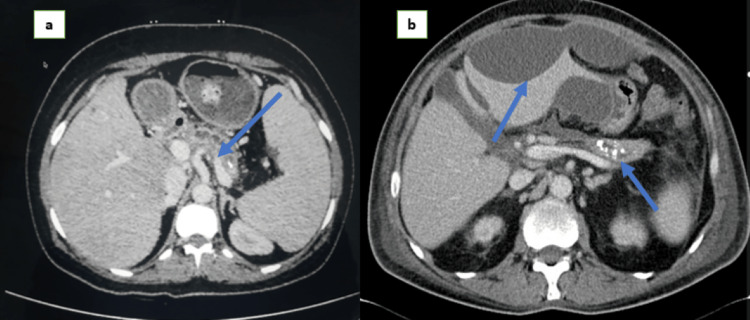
CT demonstrating atrophic pancreas with calcifications (a) seven months ago and recent CT showing well-defined cystic lesions in the left lobe of the liver with the calcified pancreas (b). CT: computed tomography
